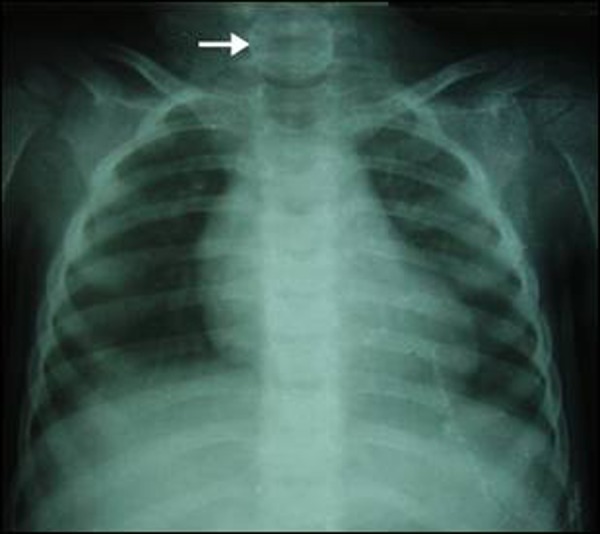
Figure 3: X-ray neck and Chest: showing faintly visible aluminum coin in the cervical esophagus (Arrow) in the 2nd patient
